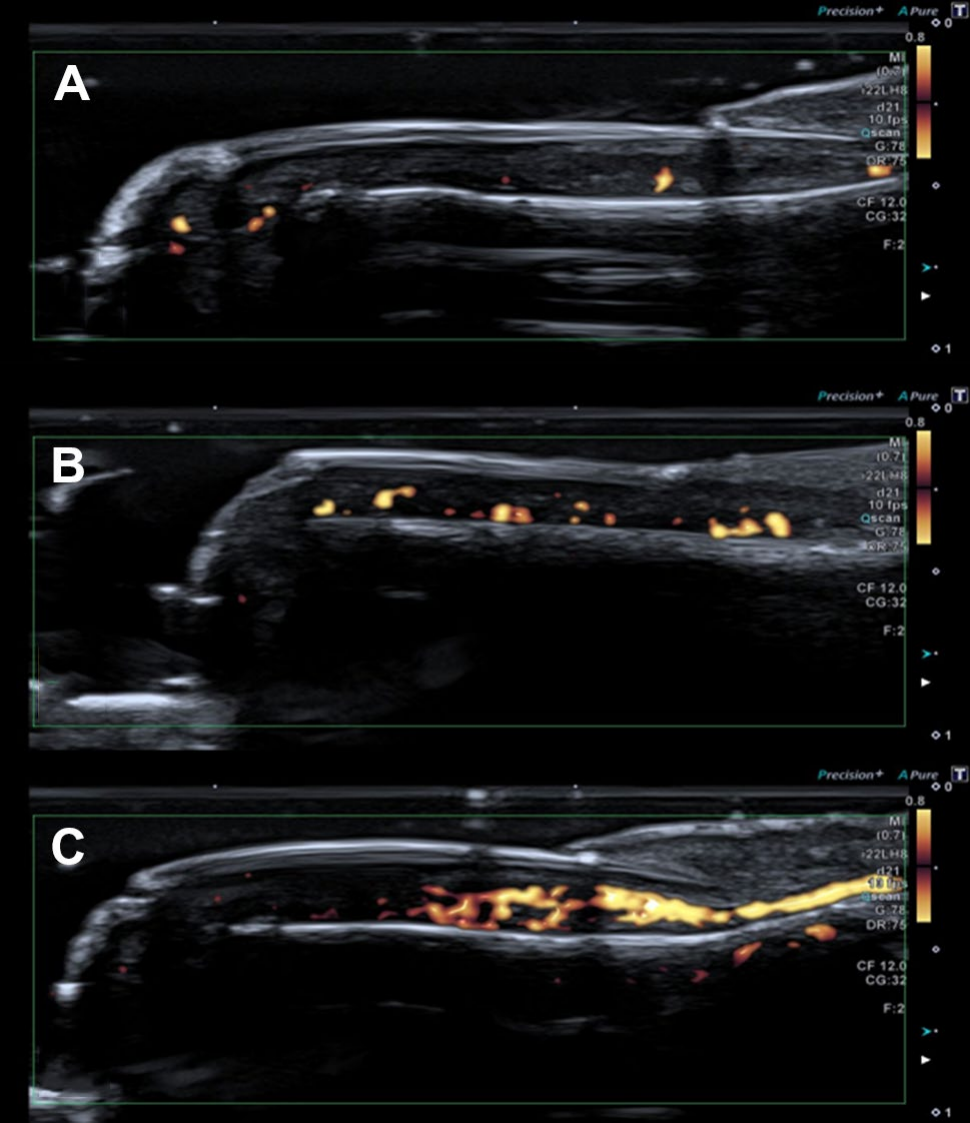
Power Doppler signal (PDUS) grading, accordingly with the classification of Gutierrez and colleagues [30]. In picture A the score is considered mild, with PDUS extension inferior of 25% of the total area of the nail bed. Two single spots and one confluent are clearly visible. In picture B several single and confluent spots are present, with moderate grade and an interested area covering less than 50% of the total. In picture C large and confluent vessels covered more than 50% of the area, configuring the PDUS as severe. Vascularization of the nail may be puzzling since three orders of vascular arcades (superficial, proximal and distal) creates anastomoses and may affect PDUS in magnitude also in healthy subjects because the vascular bed is rich in vessels
